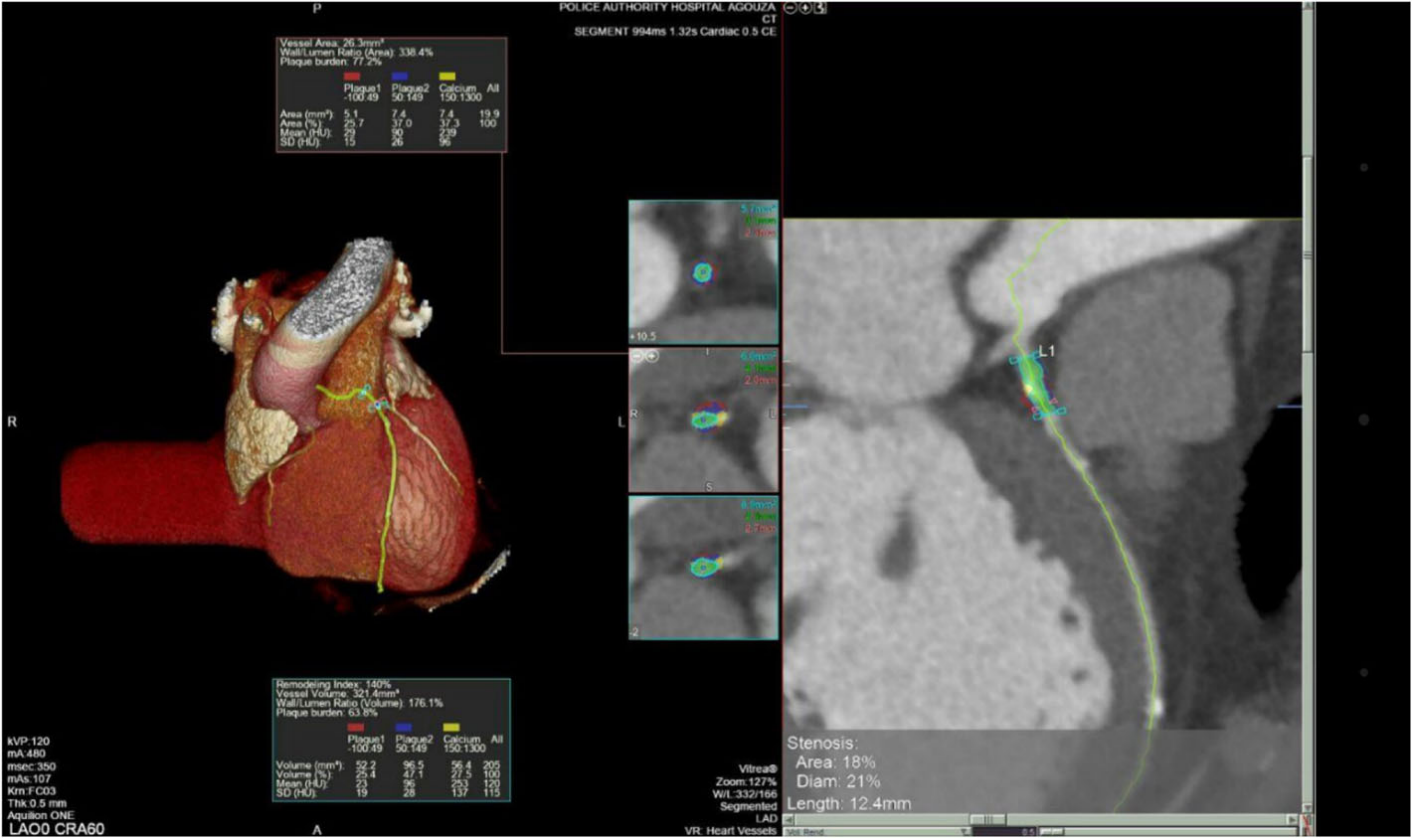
MSCT angiography coronary of a male patient, a 42-year-old smoker, and complaining of chest pain: HbA1c was 11, cholesterol level was 249, TGS 250, HDL 35, and LDL 100. CT angiography shows the proximal LAD lesion with remodeling index 1.4%, plaque burden 77.2%, wall lumen ratio 338.4%, stenosis area 18%, stenosis diameter 21%, and stenosis length 12.4 mm
